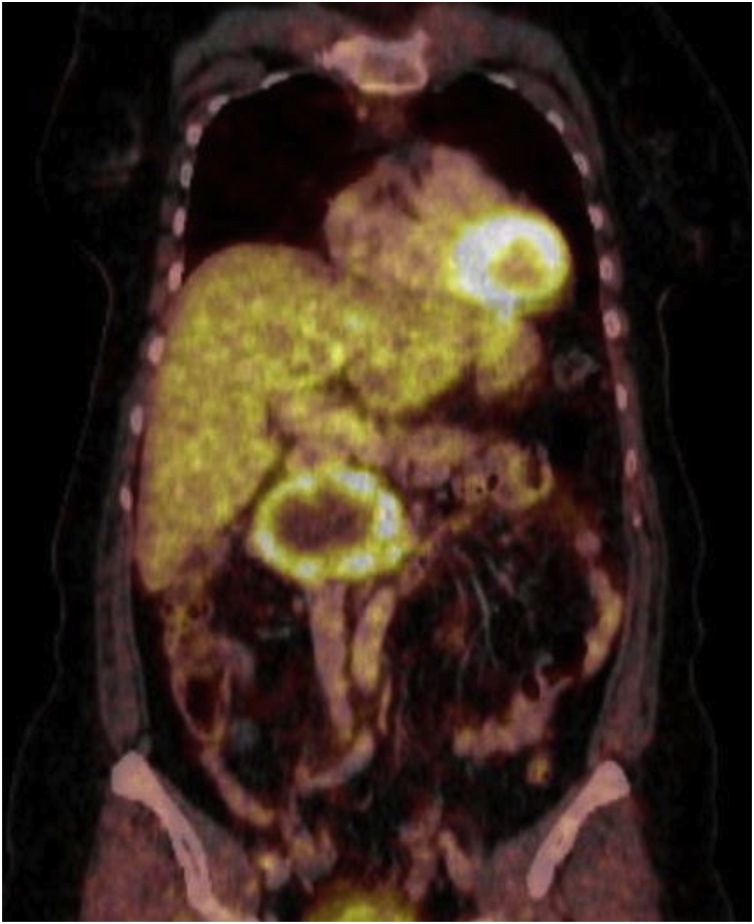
[^18^F]FDG-PET/CT confirmed the presence of a lateral space-occupying lesion para-aortal right at the level of the renal hilus with a malignoma-typical increase in metabolic activity in the marginal areas.
